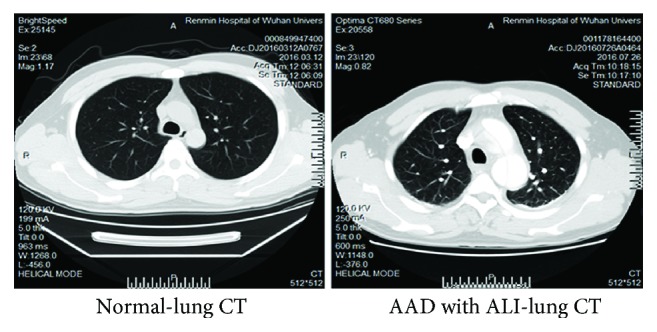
Comparison of pulmonary CT findings in patients with AAD or normal individuals. The pulmonary markings were clear in these patients with no solid shadows or exudation.
